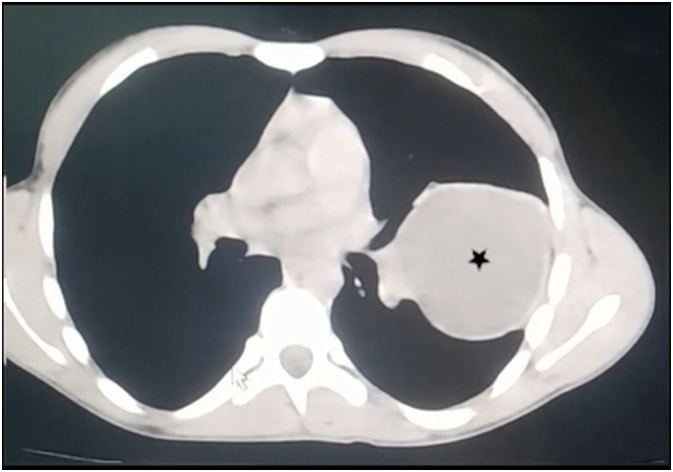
Chest CT scan showing a hydatid cyst lesion with homogenous content and smooth hyperdense walls (Black star).
